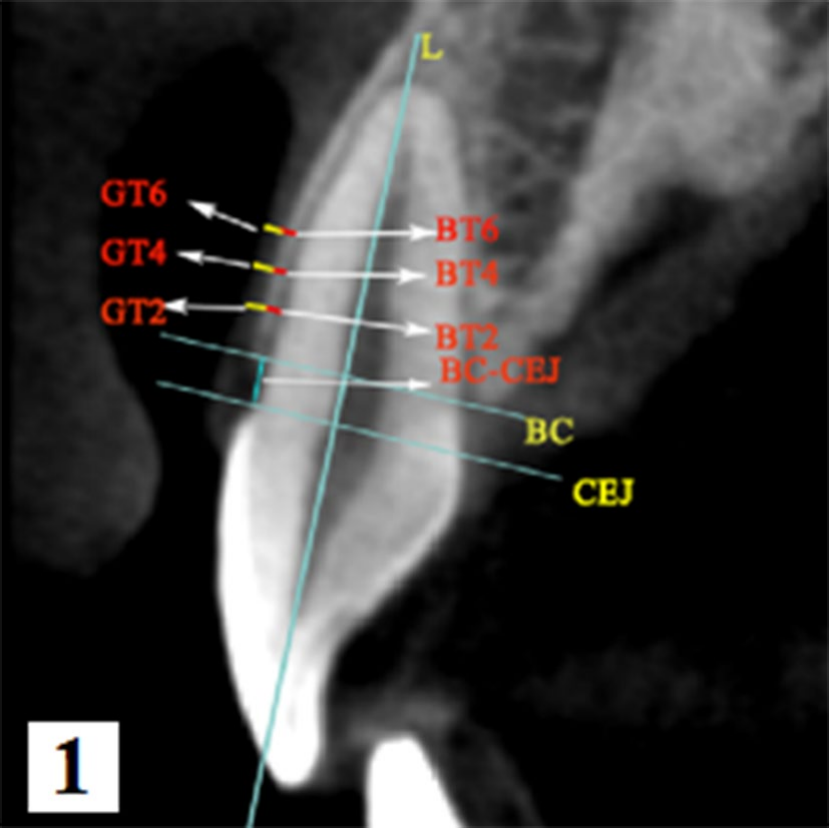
Measurements were taken in the median sagittal plane of teeth with a simple CBCT: distance from the bone crest to the CEJ (BC-CEJ); gingival thickness (GT2, GT4, and GT6) at 2, 4, and 6 mm apical to the CEJ; labial bone thickness (BT2, BT4, and BT6) at 2, 4, and 6 mm apical to the CEJ.
